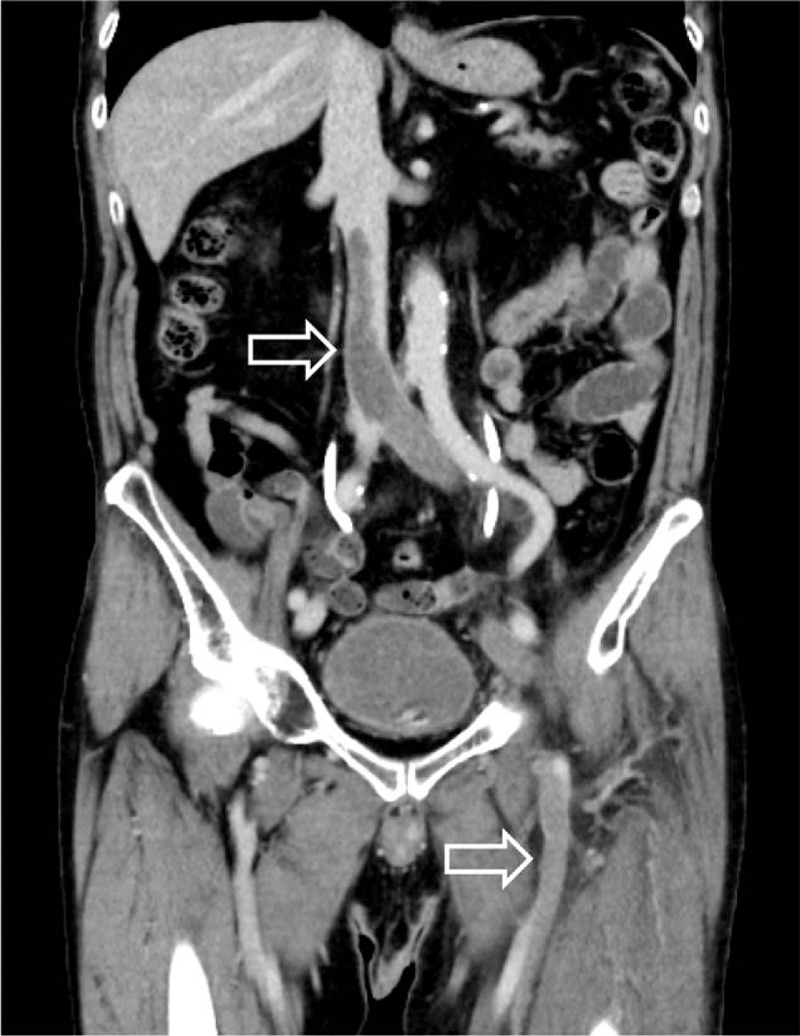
Abdominal computed tomography scan on admission showing a massive thrombus in the inferior vena cava and iliofemoral vein (arrow).
